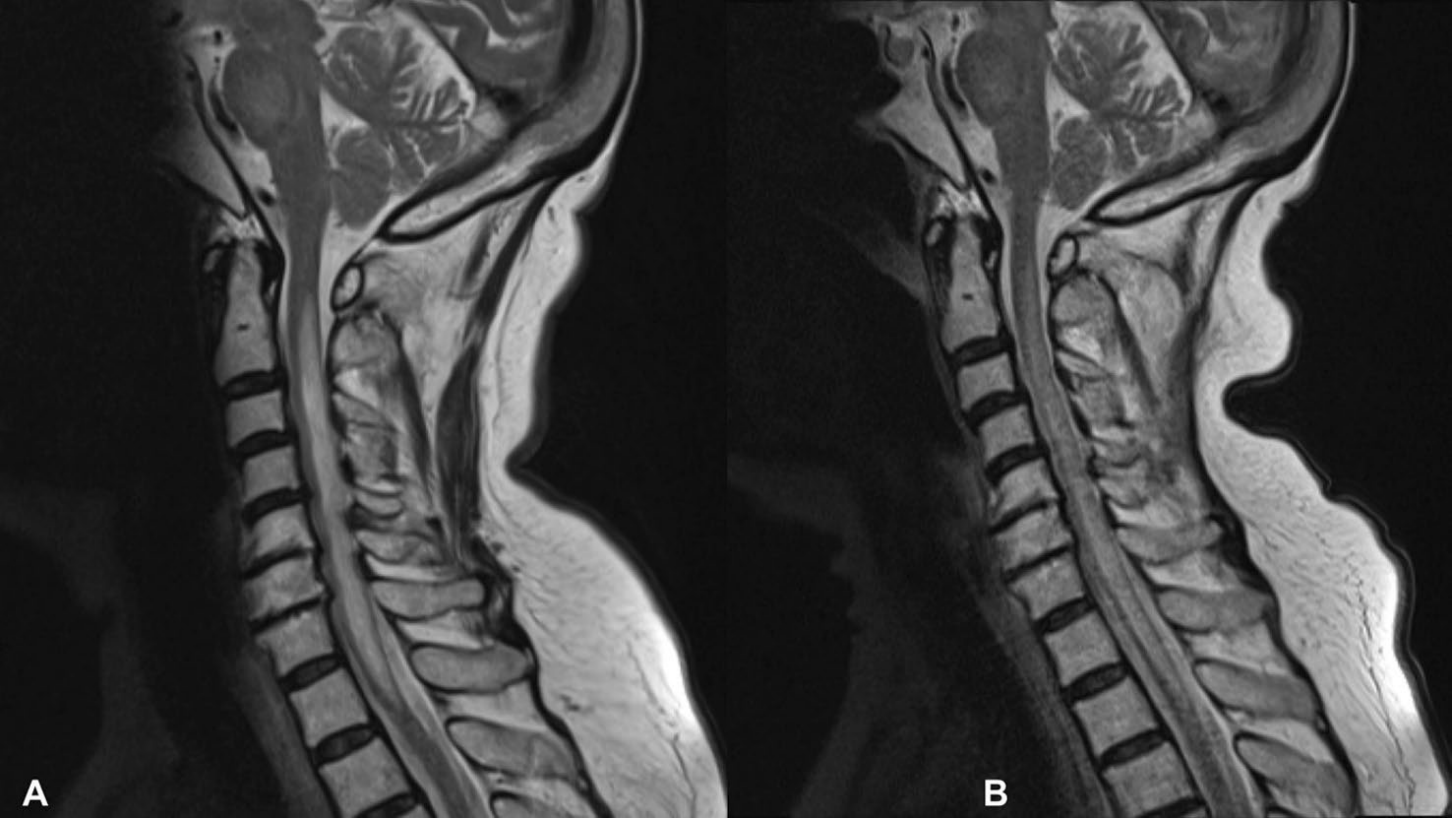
Sagittal T2-weighted MRI at 1.5T demonstrating the LETM extending from the C1 to T1 level on admission (A) and longitudinal progression 7 days later despite therapy, reaching the level of the lower vertebral body endplate of T2 (B)
